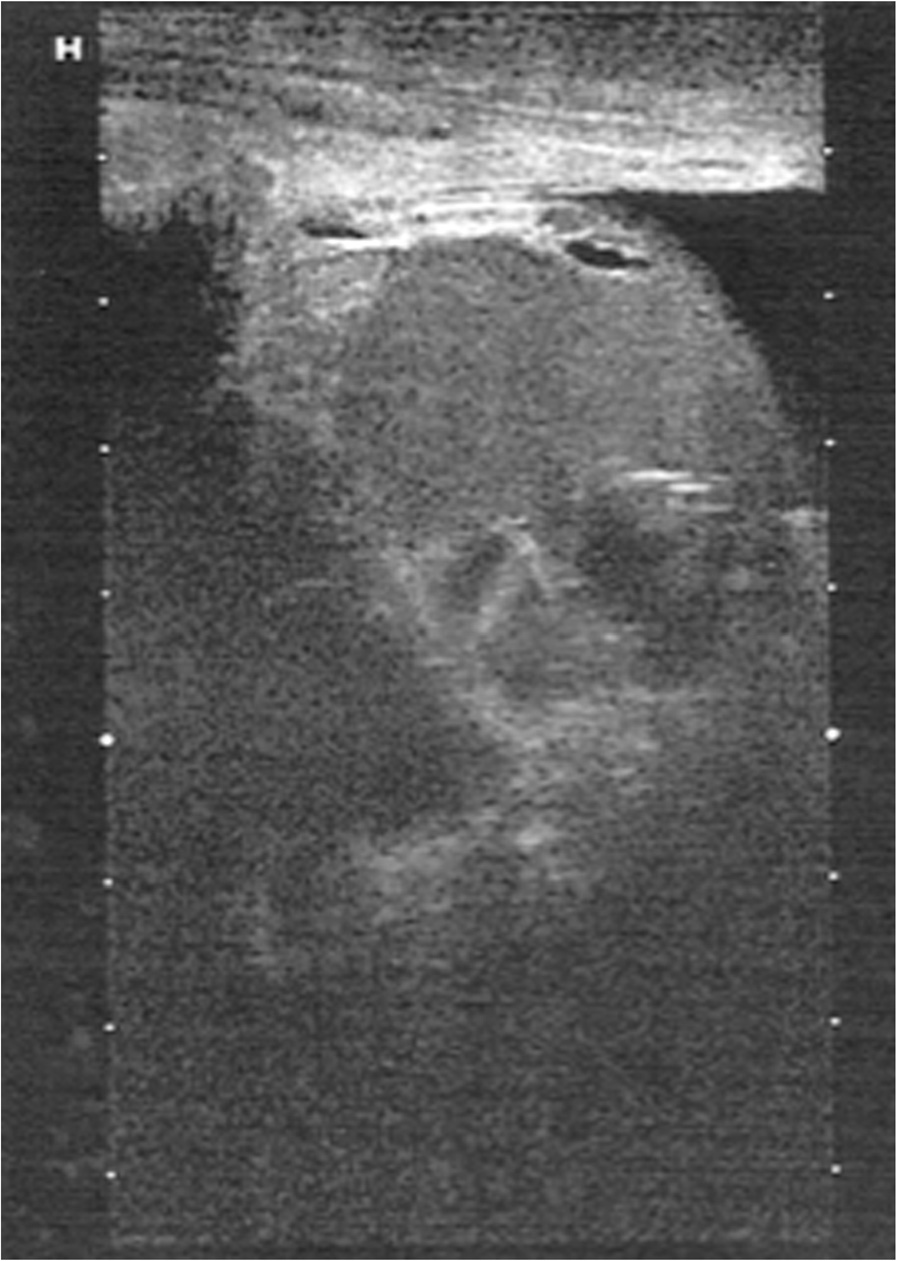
Ultrasound view of a partly cystic tumor.
